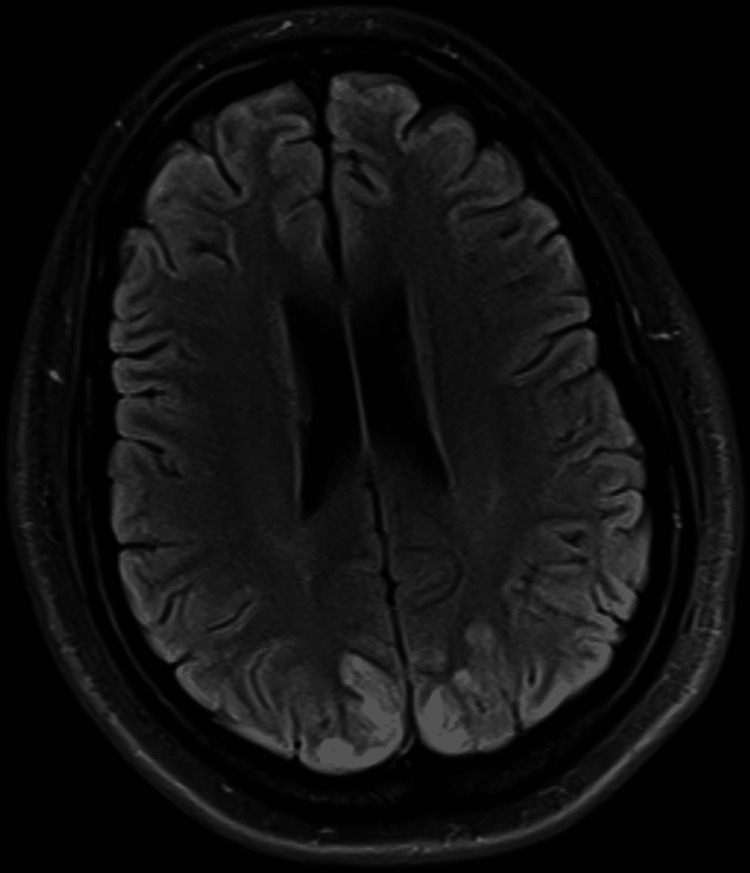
MRI demonstrating increased T2 and FLAIR signal in cortical and subcortical regions of the parietal and occipital lobes.
